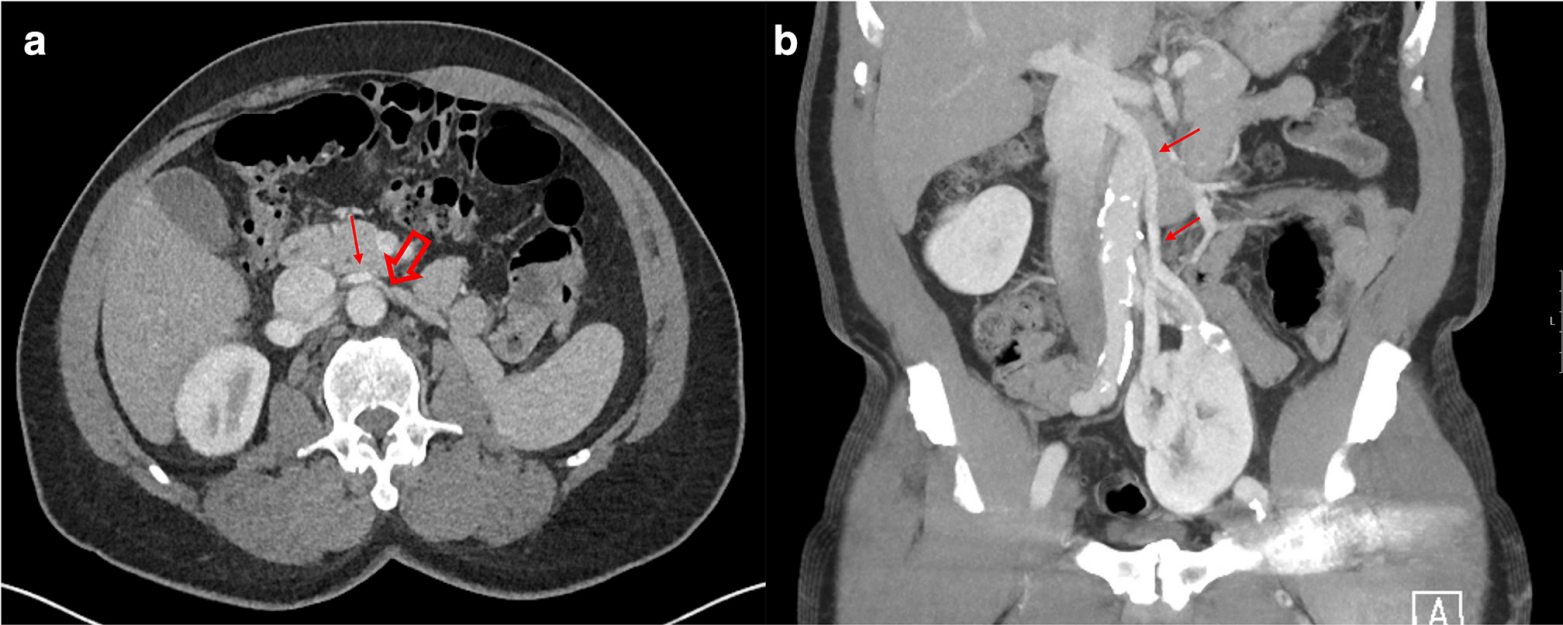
(a) Axial image of portovenous CT at the level of L2. Solid red arrow demonstrating the left lower pole renal vein branch passing anterior to the aorta. Open red arrow demonstrating a small branch arising from the left lower pole renal vein thought to be a candidate for the left testicular vein. (b) Coronal reformat demonstrating the course of the lower pole renal vein.
